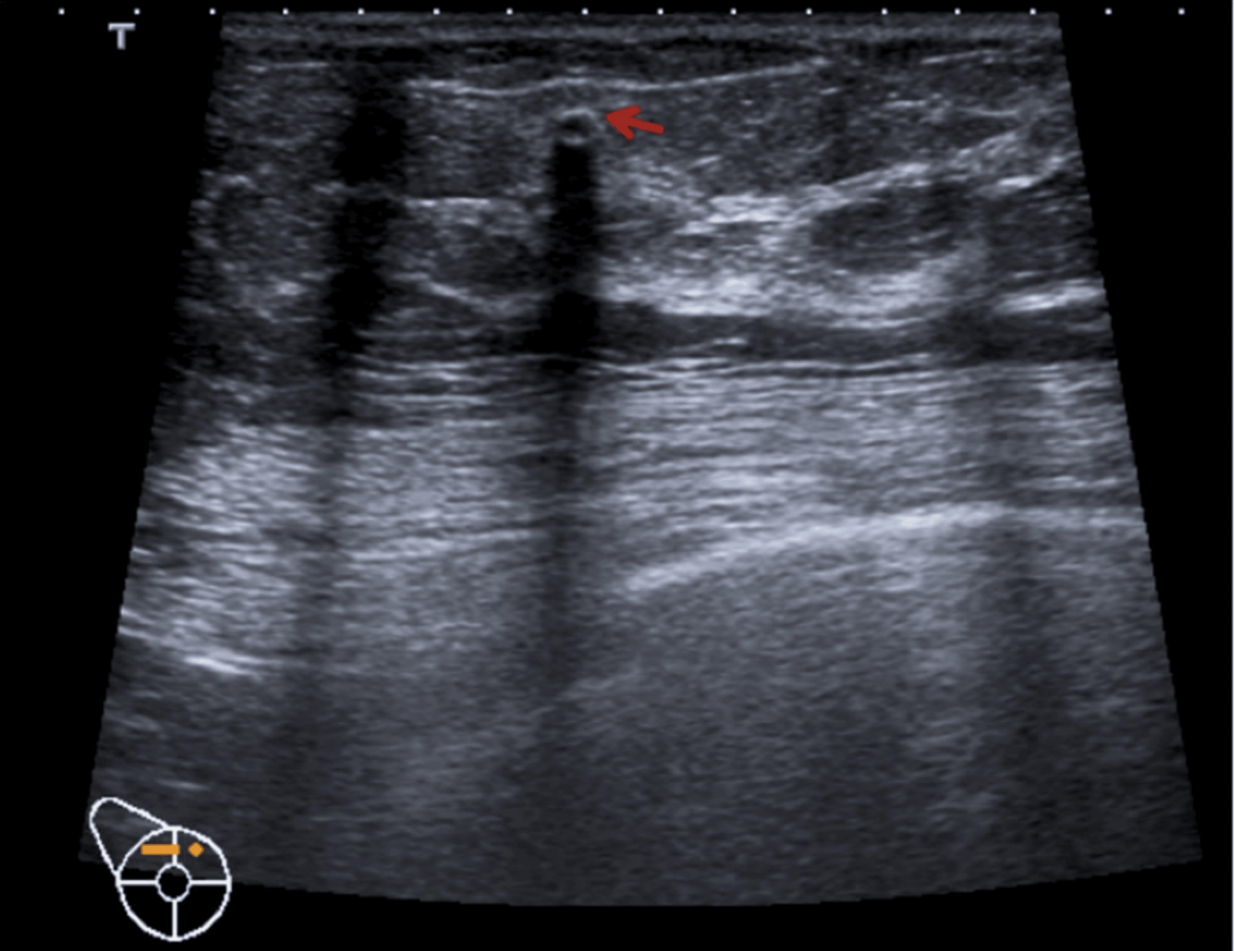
Breast ultrasound indicating fibrotic changes in the glandular tissue and a coarse calcification (red arrow) Image credit: Magalhaes et al. [43] (licensed under Creative Commons Attribution-NonCommercial-ShareAlike 4.0 International License; http://creativecommons.org/licenses/by-nc-sa/4.0/)
